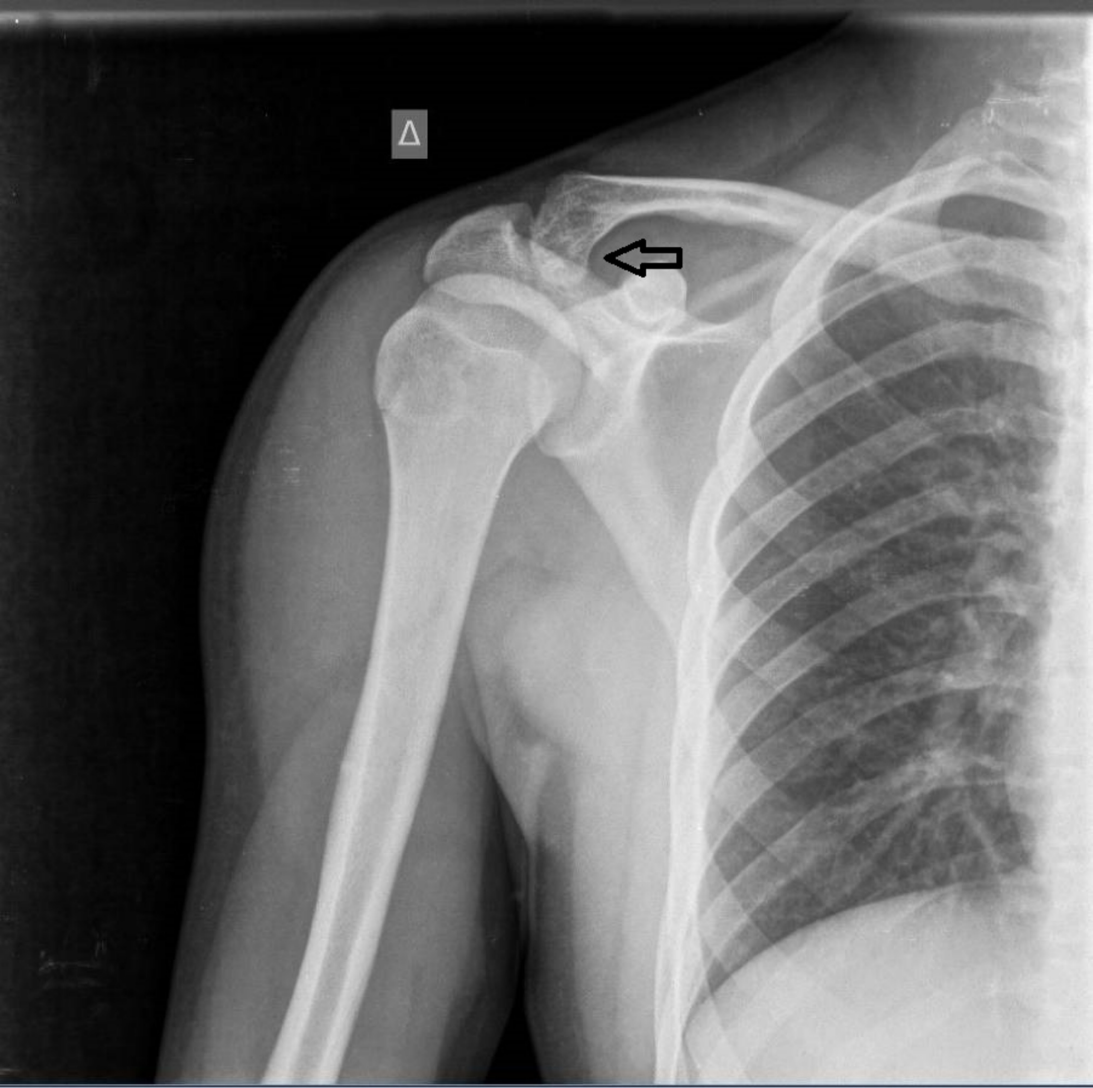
Anteroposterior radiograph of the right shoulder. A distal clavicle tumor is depicted.
